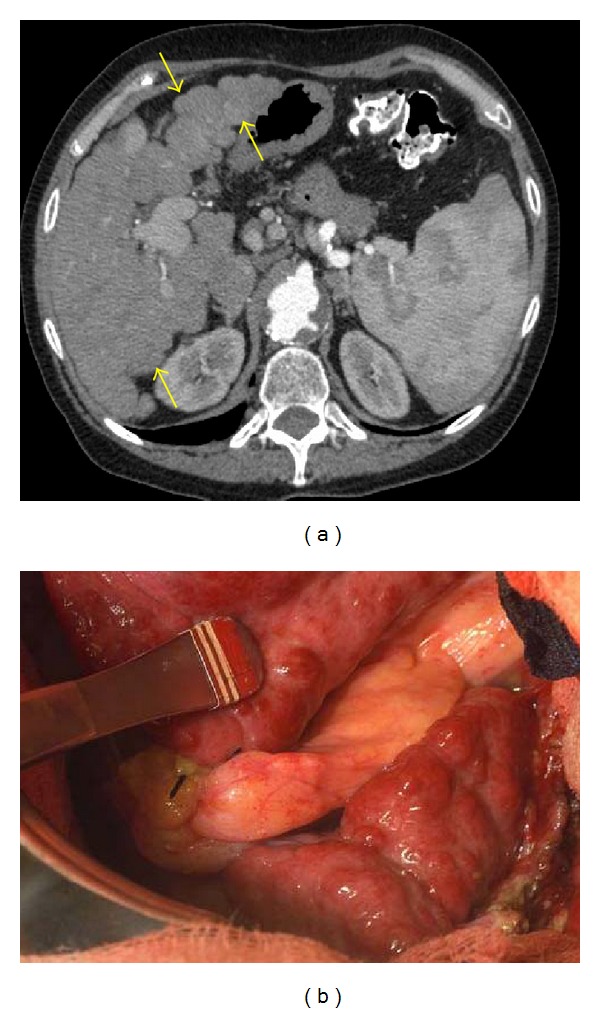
Macronodular regenerative nodules, due to alcohol-induced cirrhosis. (a) Arterial phase CT shows multiple nodular isodense lesions deforming the liver margin (arrows). The contour bulge caused by the nodular regeneration may help to detect the lesions. (b) Intraoperative photograph of macronodular cirrhosis.
